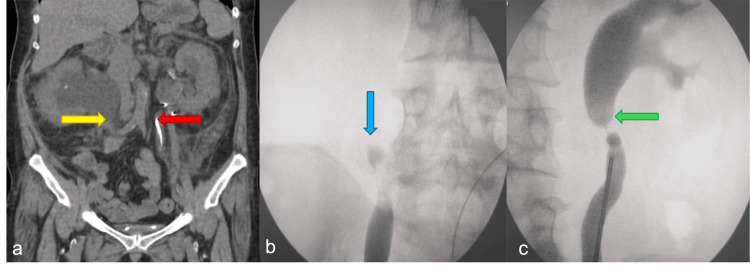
CT image (a) and fluoroscopic images of both kidneys (b, c) (a) Right-sided pelvi-ureteric junction obstruction/stricture(yellow arrow). (b) Blind ending ureter with no contrast passage across the narrow segment observed on the right side (blue arrow). (c) Narrowing at the L3 level of the ureter on the left side (green arrow).
